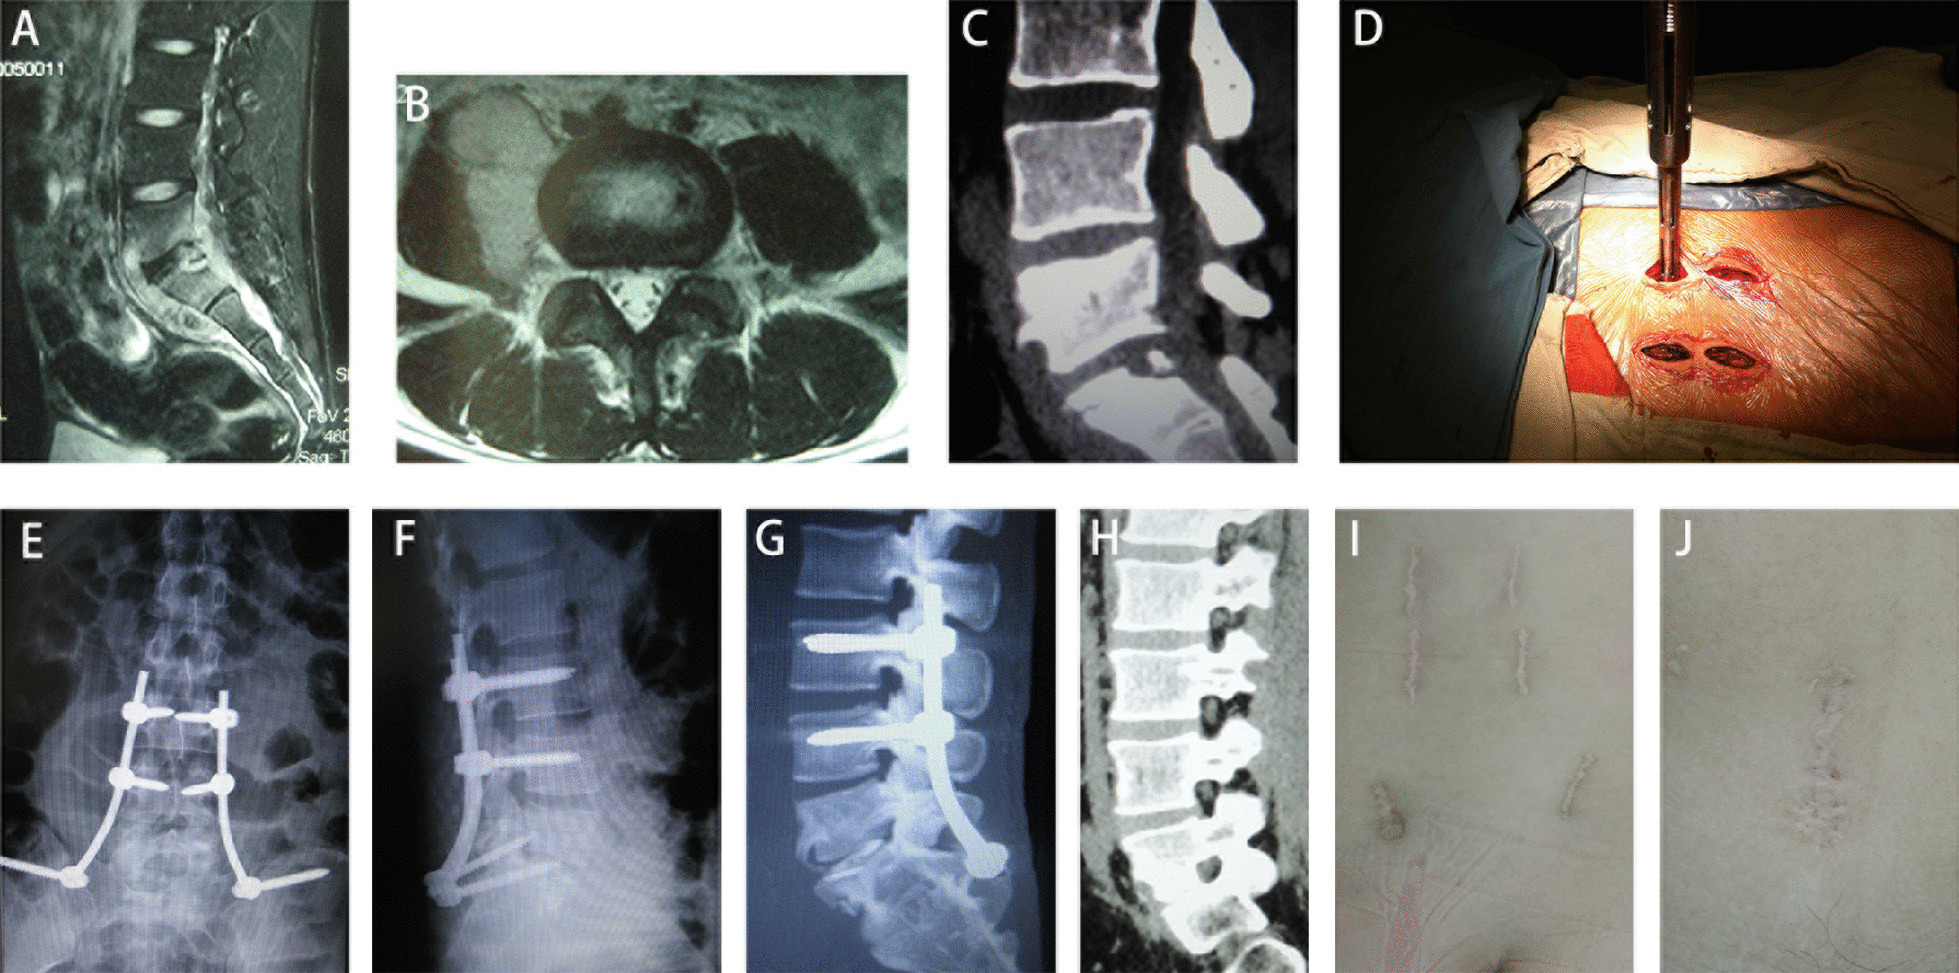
A 20-year male patient with L5–S1 tuberculosis accompany with psoas abscesses. Preoperative MRI (A, B) and CT (C) showed destruction of L5–S1 and psoas abscesses. Freehand MIPS and minimally invasive iliac screws (D). Postoperative x-ray (E, F) and CT (G) showed good position of the autogenous iliac bone and internal fixation. CT at 12-month after surgery (H) showed bone fusion achieved. Surgical incisions of freehand MIPS and iliac screws fixation (I) and OLIF (J)
